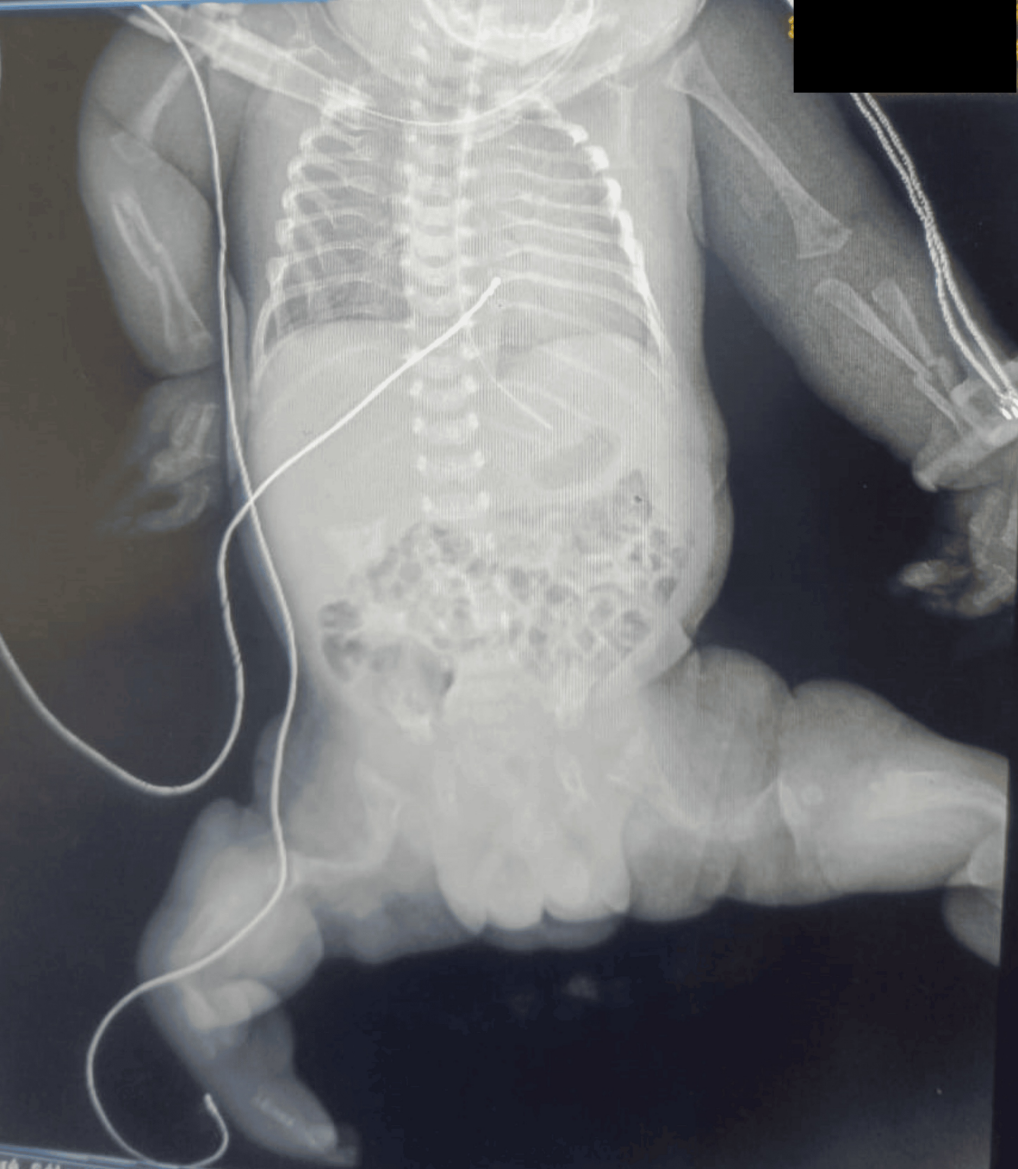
Full-body frontal radiography in supine position. Multiple bilateral fractures of the radius and ulna, accompanied by severe bone deformities and pronounced bowing, predominantly affecting the femurs and tibias. Of particular note is the presence of a malunited fracture in the right tibia.
